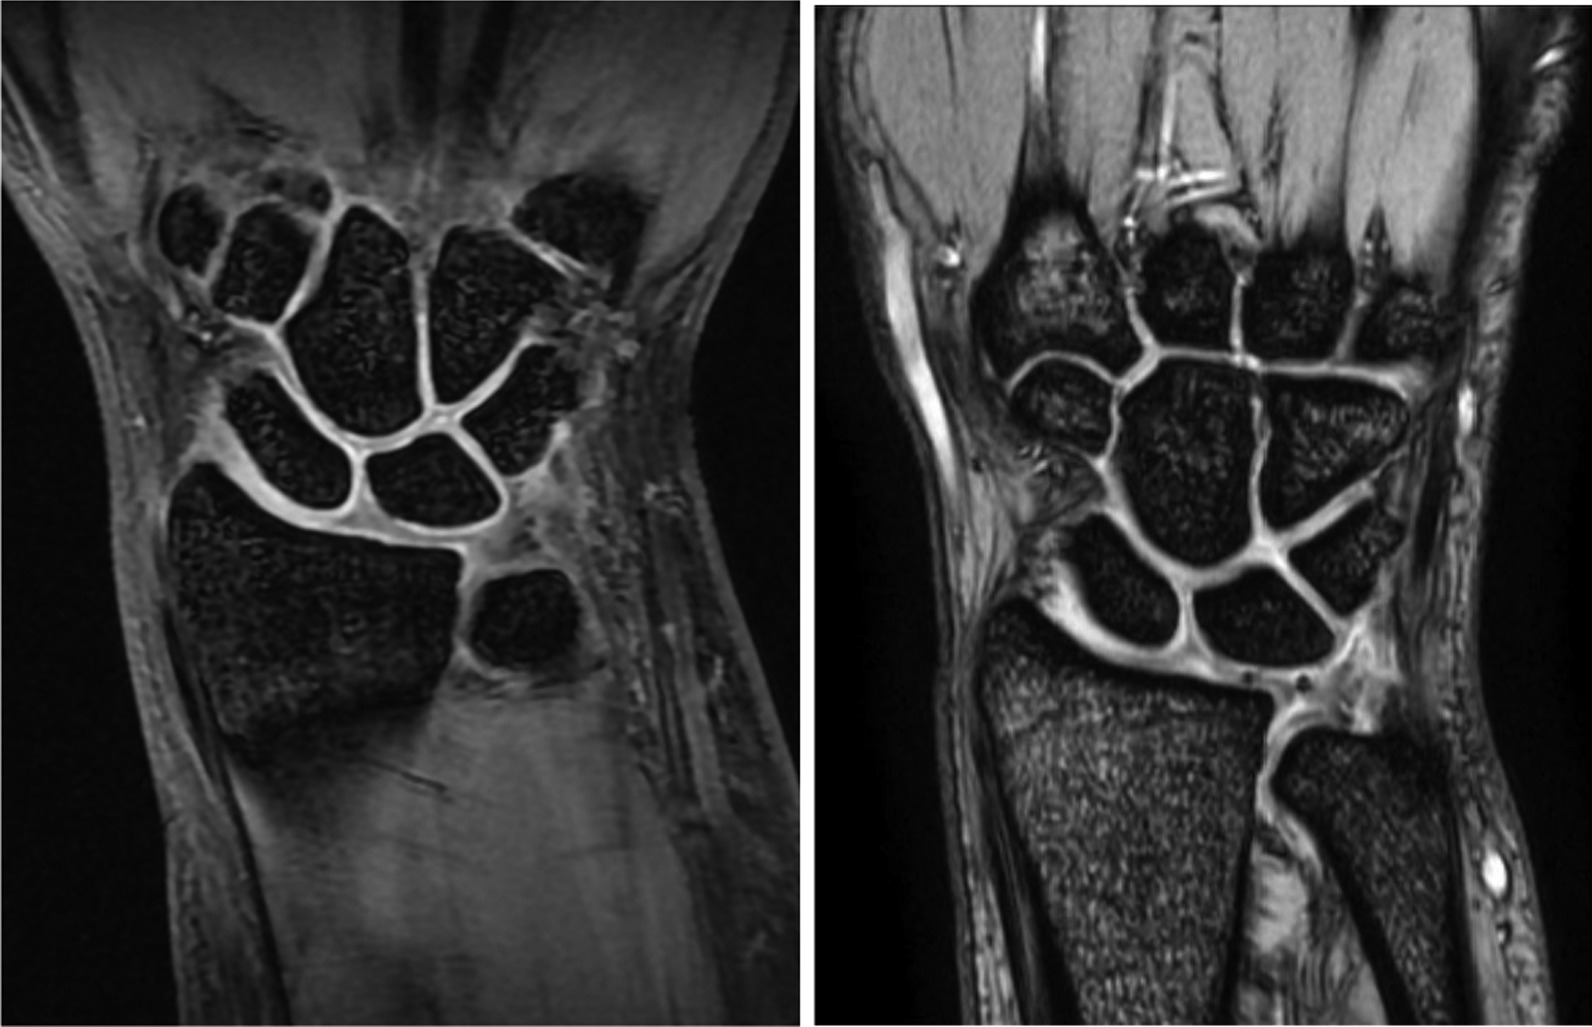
A MRI view example of a patient before (left) and after (right) their all-inside arthroscopic suture anchor surgical procedure
